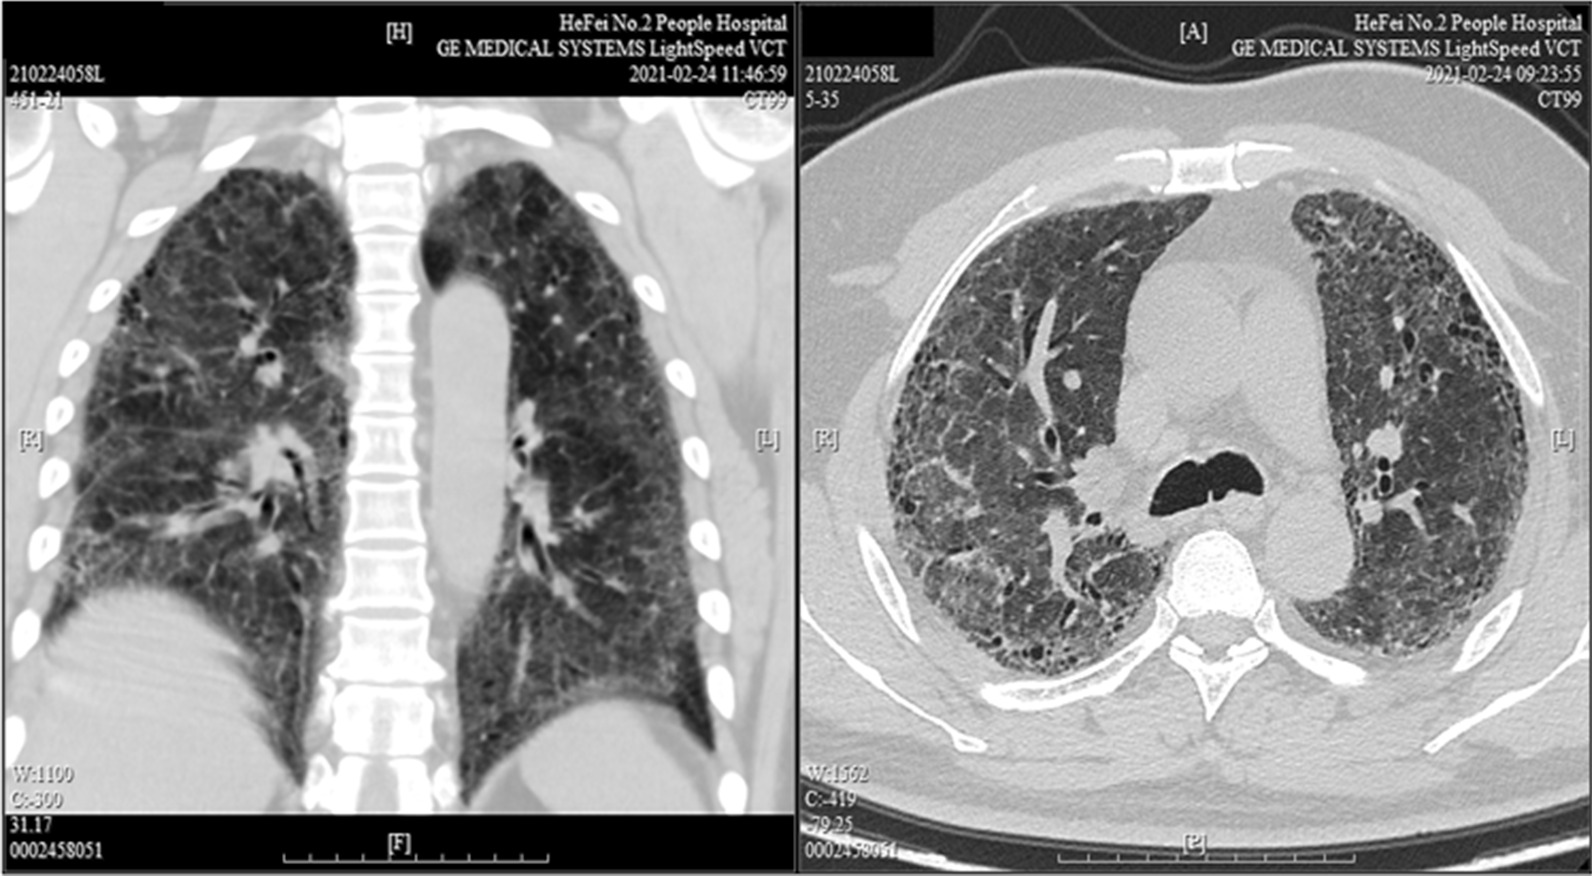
Chest CT suggesting bilateral inflammation in the lungs
